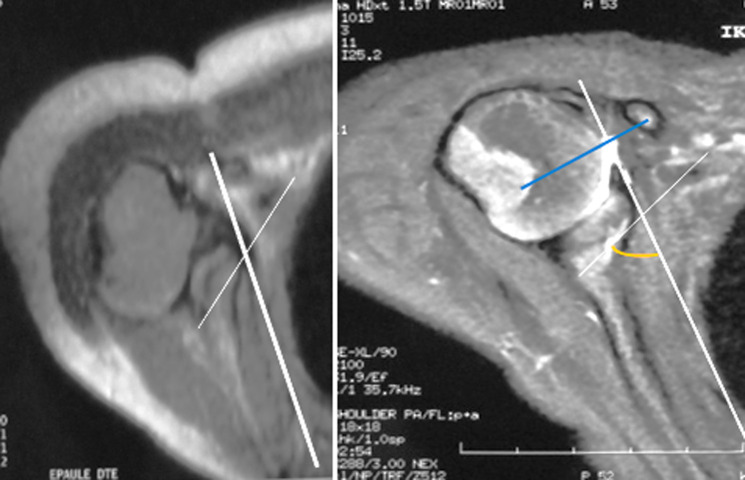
preoperative and 5 years postoperative MRI in a 3-year-old female with right residual brachial plexus palsy
